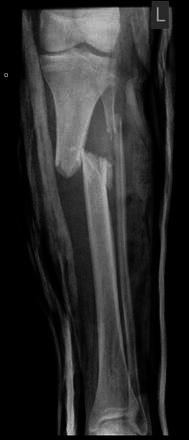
Preoperative radiograph of an unstable proximal 1/3 tibial fracture
